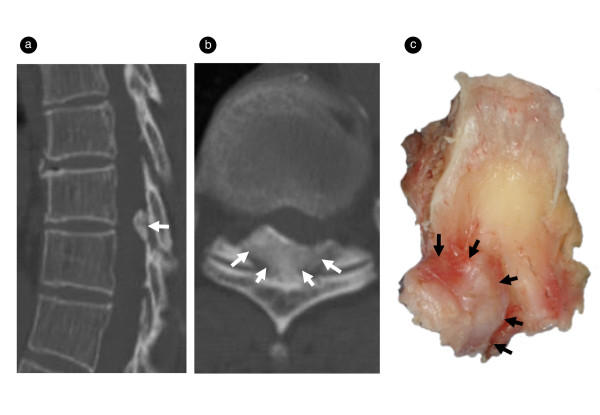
Representative case of tuberous type OLF. Sagittal (a) and axial (b) CT images obtained in a 72-year-old woman with T11-12 OLF. Note the fusing of the ossified plaque at midline and the progression of this process into the spinal canal. The resected sample (c) contains a hard mass from dura mater aspect (arrow, ossification). CT, computed tomography; OLF, ossification of the ligamentum flavum.
